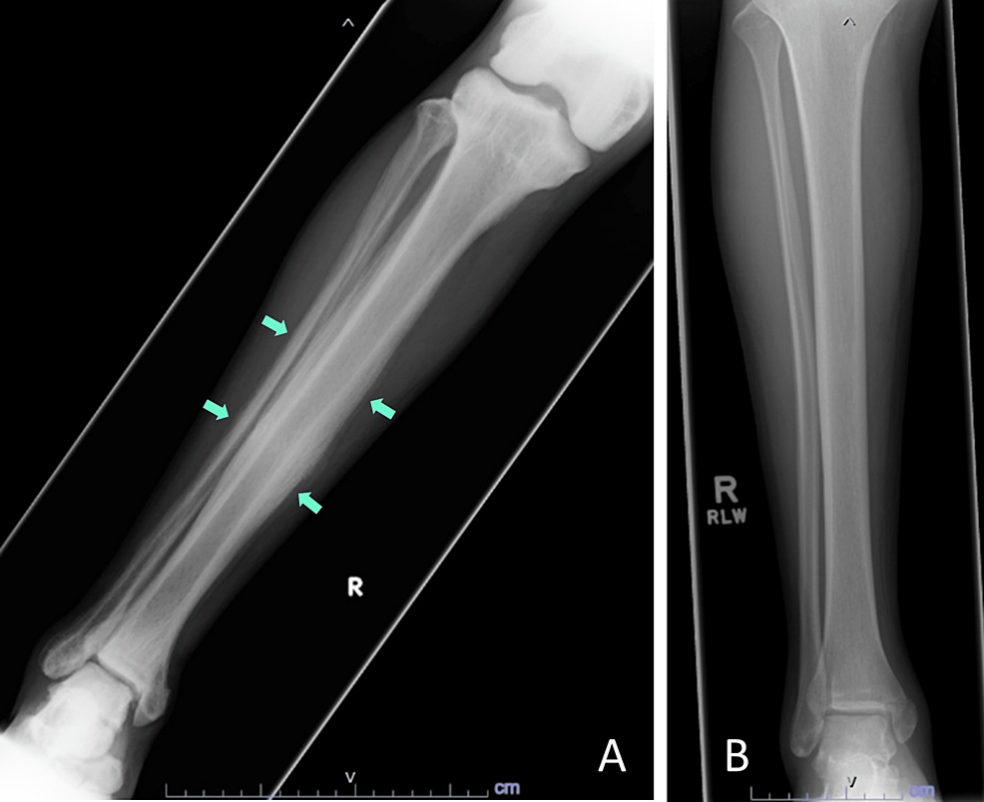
X-ray of the right tibia and fibula (A) showed diffusely increased sclerosis and cortical expansion, while an X-ray from two years prior (B) demonstrated only minimally displaced transverse fracture through the medial malleolus after a motor vehicle accident; no sclerosis or cortical expansion of bones were observed at that time.
